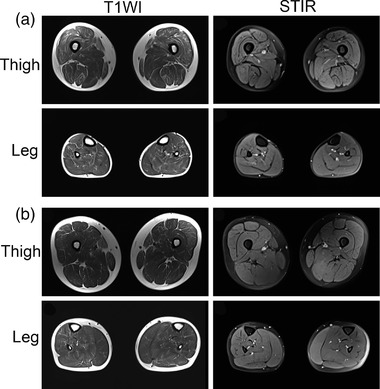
Muscle MRI changes of lower limb in patient one (a) and patient two (b) with *GFPT1*‐related CMS. Thigh level showed a slightly diffused hyperintensity on T1WI except adductor magnus and semimembranosus muscles; leg level showed a slightly diffused hyperintensity except medial gastrocnemius that simultaneously had mild high‐signal on STIR
